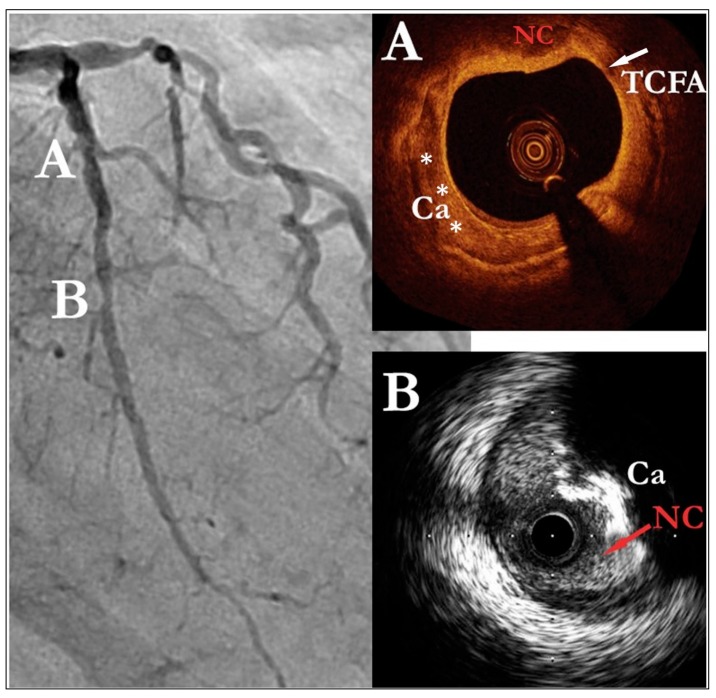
Images of intracoronary imaging modalities. A: Plaque morphology by optical coherence tomography. B: Intravascular ultrasound images. TCFA = thin-cap fibroatheroma; Ca = calcifications; NC = necrotic core.
